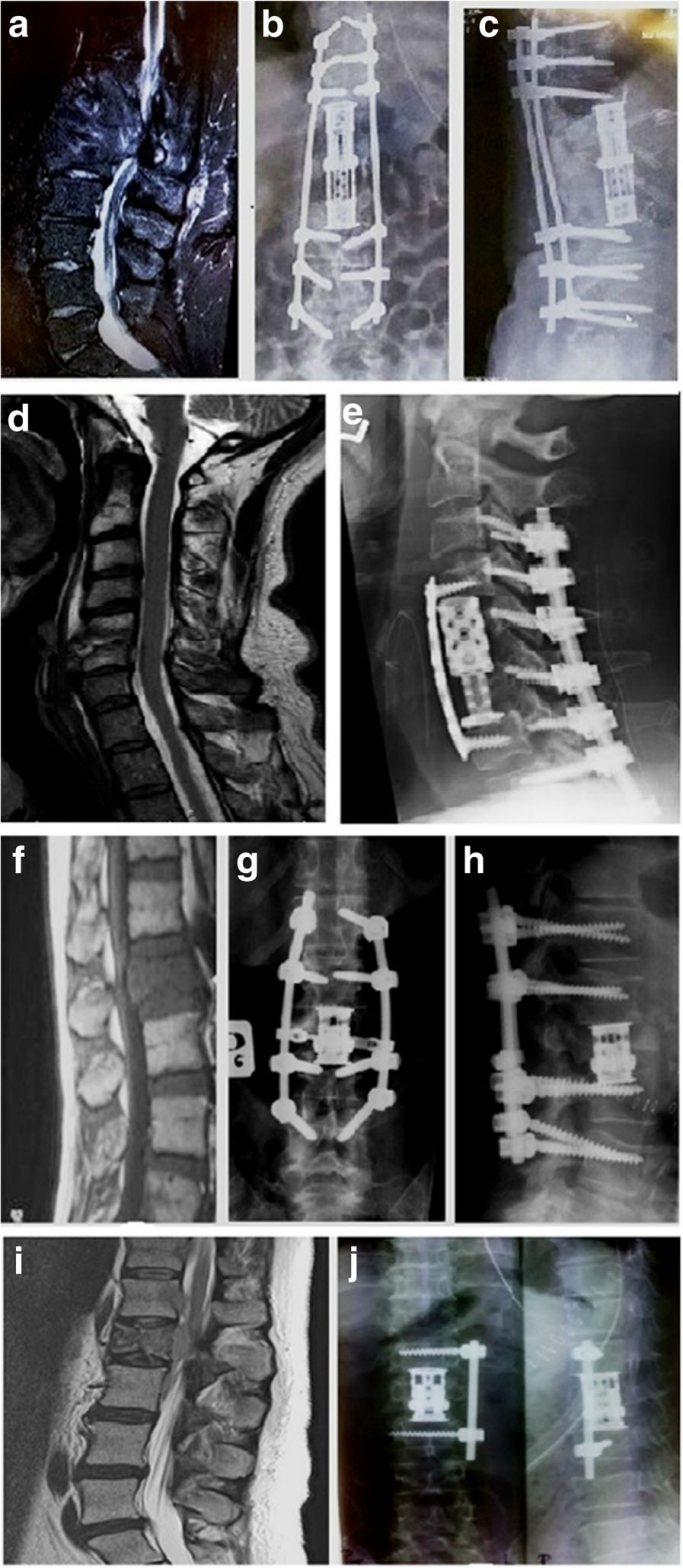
MRI image of metastatic thyroid cancer located in thoracic 11–12 and lumbar 1 vertebrae (a). Postoperative radiograms (b, c) showing vertebral prosthesis and posterior spine fixation. MRI images of C5-C6 breast cancer metastasis (d), and after tumor resection and cervical spine reconstruction (e). MRI image of metastatic breast cancer in the second lumbar vertebrae (f). Postoperative radiograms (g, h) after 360 degree reconstruction of the spinal column. MRI of fractured second lumbar vertebrae (i) and after prosthesis implantation and lateral spinal fixation (j)
